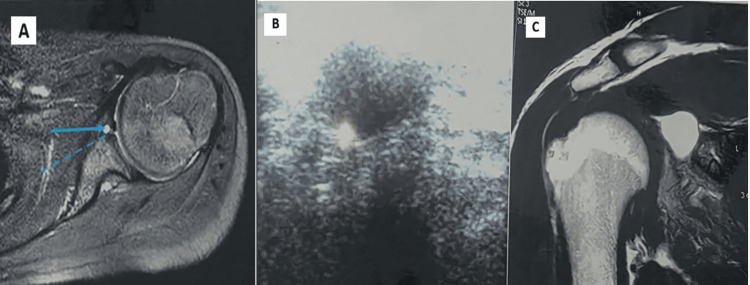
Labral cyst: Axial PDFS MRI image showing a tiny cystic lesion (solid arrow) along the anterior labrum with linear hyperintensity (dotted arrow) in the anteroinferior labrum, suggesting a labral tear. Paralabral cyst (B-C): (B) USG showing a well-defined, rounded cystic lesion in the paralabral region beneath the supraspinatus toward the suprascapular notch. (C) T2-weighted coronal MRI image demonstrating a paralabral cyst (arrow) arising from a labral tear (not shown) USG, ultrasonography; MRI, magnetic resonance imaging; PDFS, proton density-weighted fat-saturated
